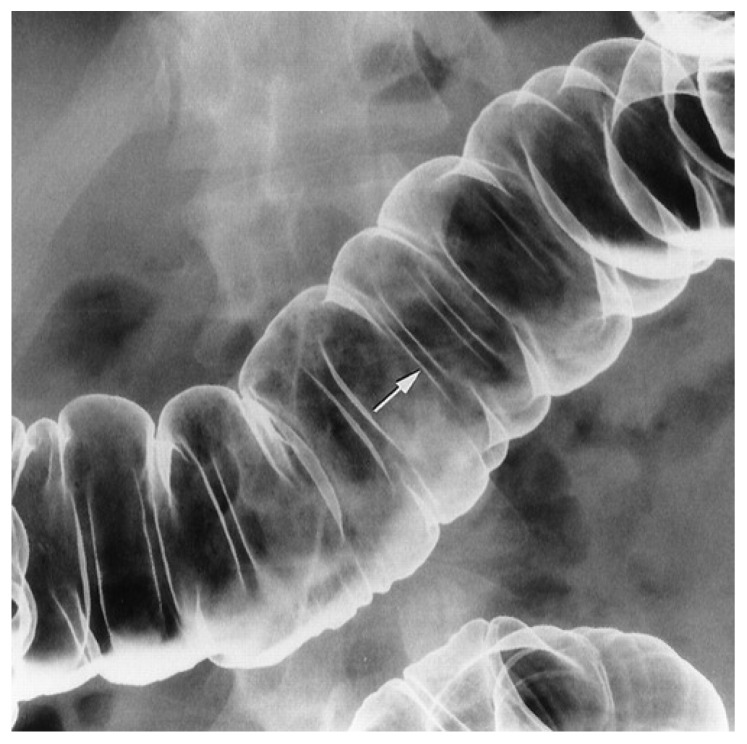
Spot radiograph of the middle of the transverse colon obtained from a patient near-erect position. The interhaustral folds are straight; a representative fold is identified with an arrow. The haustral sacculations are distended, but not overdistended and flattened. Reprinted with permission of [135].
